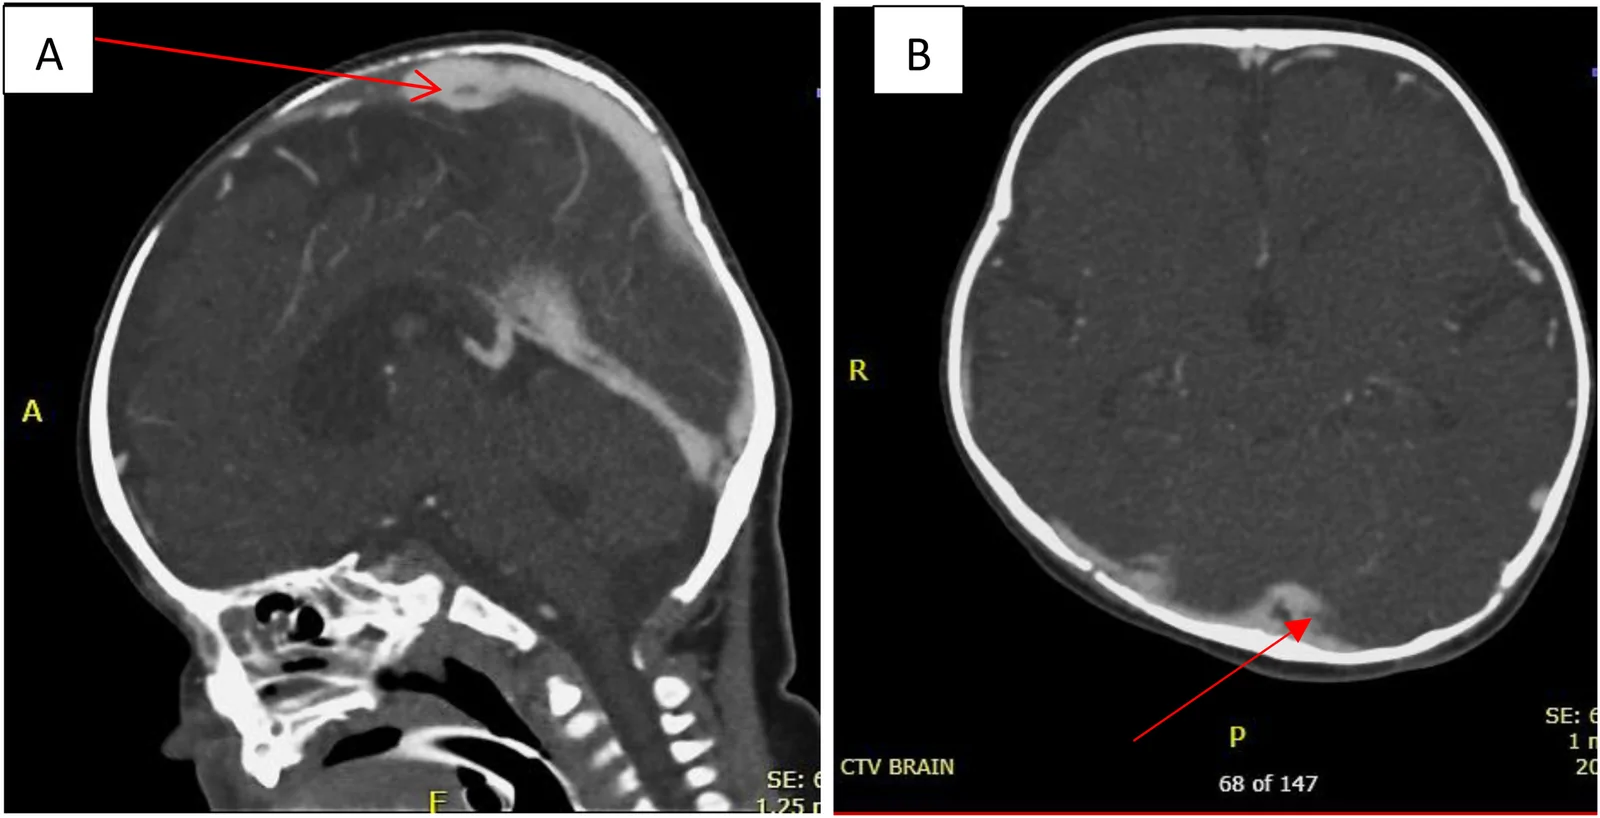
Brain computed tomography venogram (CTV) showing partial non-occlusive filling defect seen within the sinus confluence, straight sinus and left transvers sinus denoting sinus dural venous thrombosis. A; sagittal and B; axial plane.
